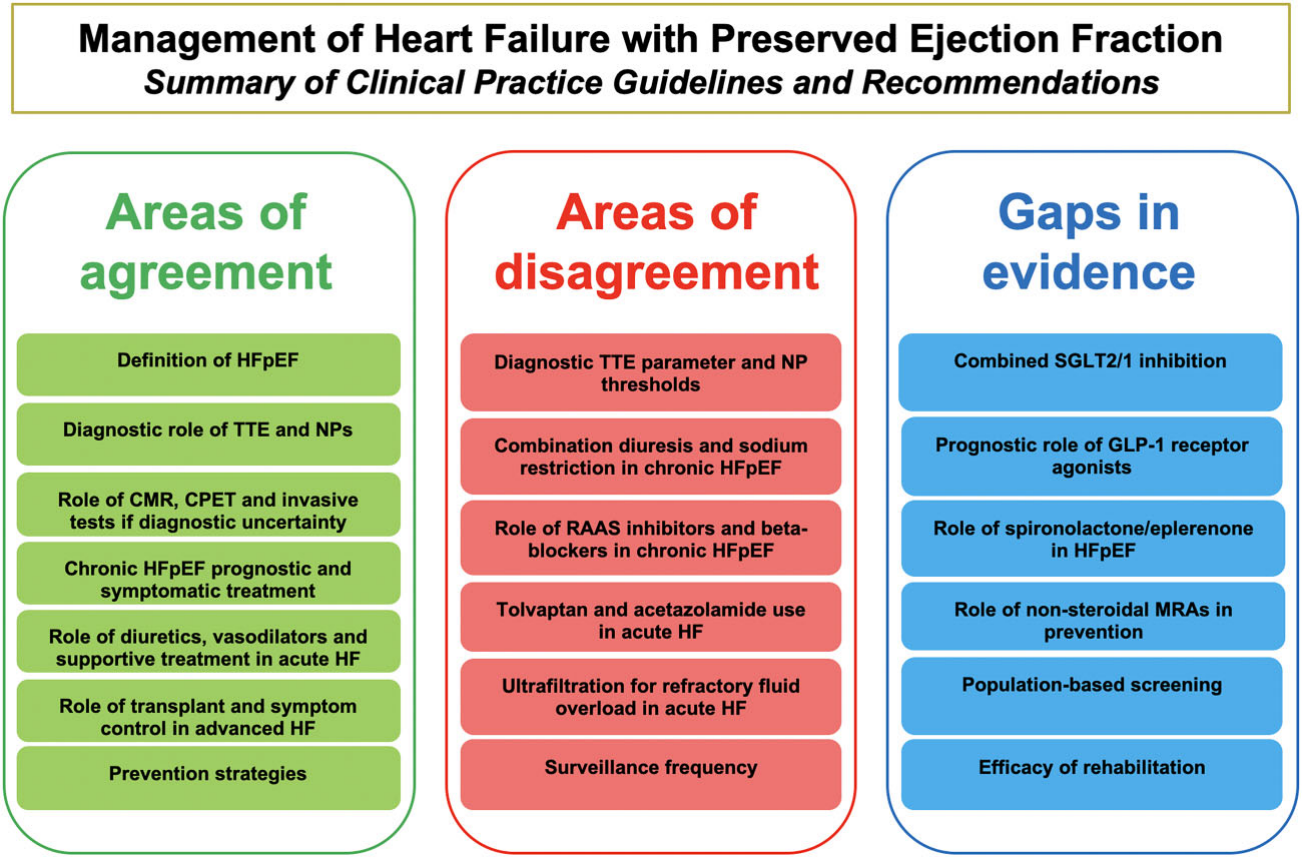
Summary of clinical practice guidelines and recommendations on heart failure with preserved ejection fraction management. CMR, cardiovascular magnetic resonance; CPET, cardiopulmonary exercise testing; GLP-1, glucagon-like peptide-1; HFpEF, heart failure with preserved ejection fraction; MRA, mineralocorticoid receptor antagonist; NP, natriuretic peptide; RAAS, renin-angiotensin-aldosterone system; SGLT2/1, sodium-glucose co-transporter 2/1; TTE, transthoracic echocardiography.
